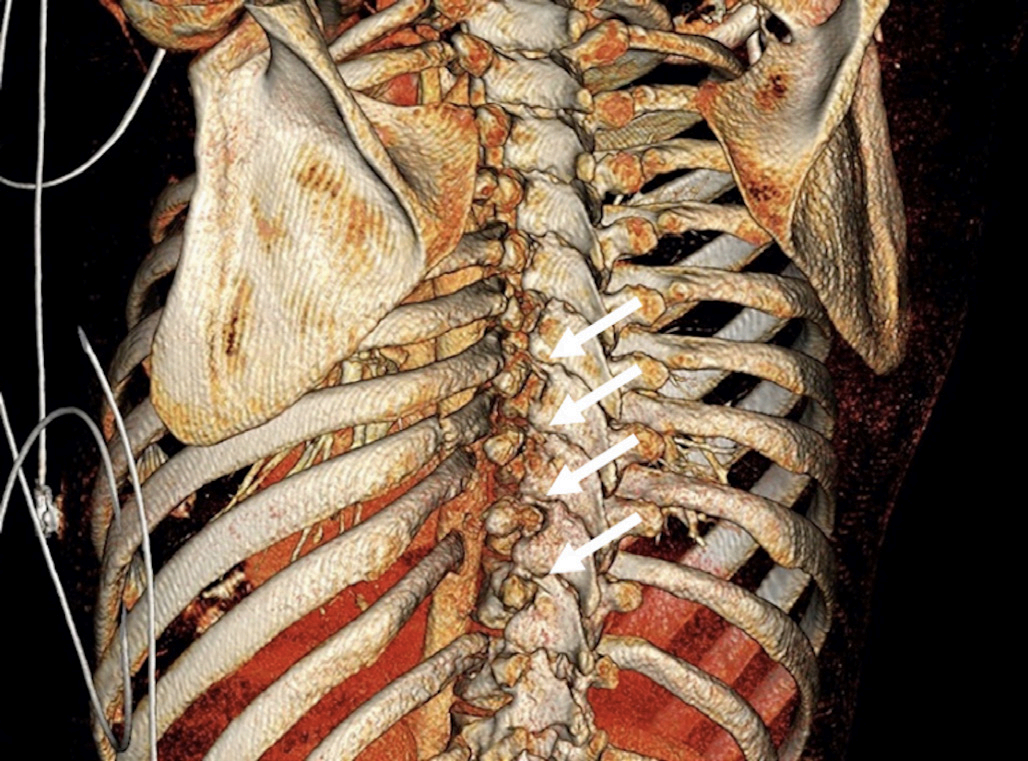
Coronal three-dimensional CT-scan. The arrows show the costovertebral dislocation of rib 7–10 on the left side. Fractures of the left rib 3–8, 11 and 12 can also be seen.
